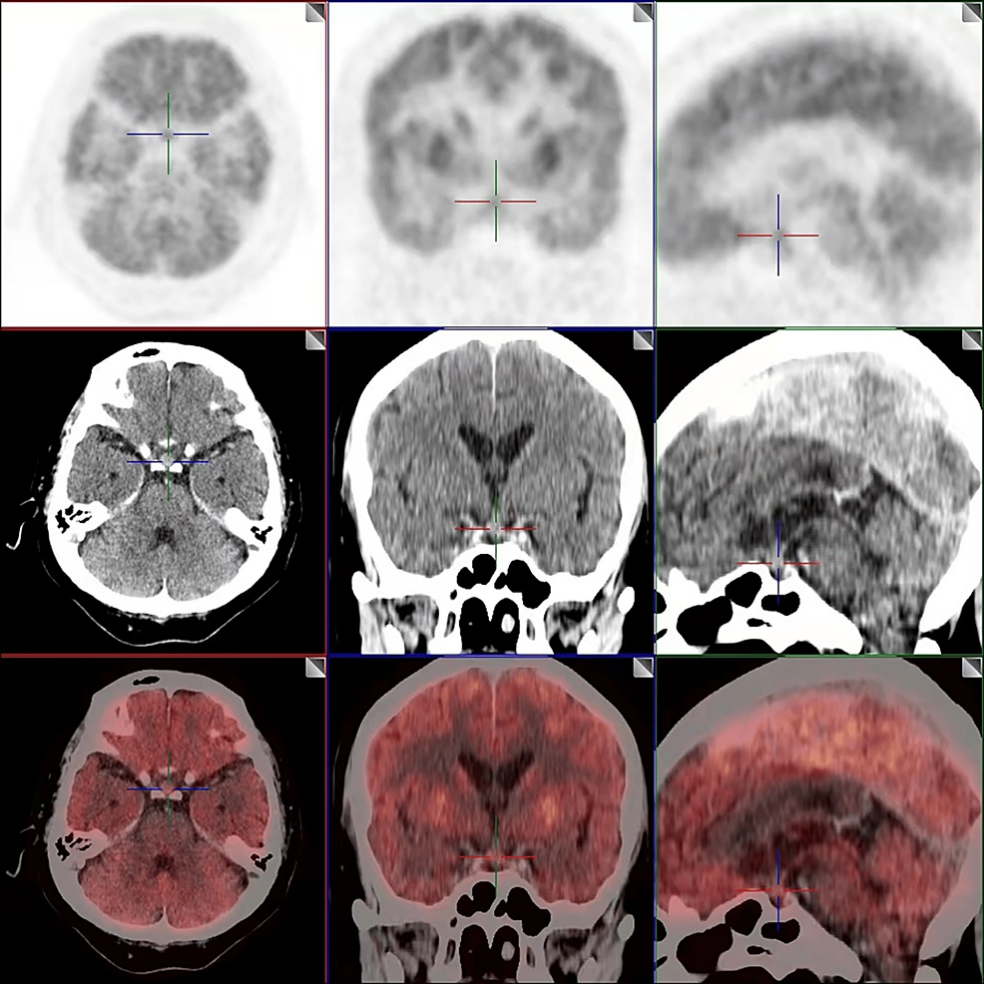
Brain PET/CT imaging of the patient indicated thickening of the pituitary stalk with increased radioactive uptake, along with slightly increased uneven radioactive uptake of the pituitary gland.
